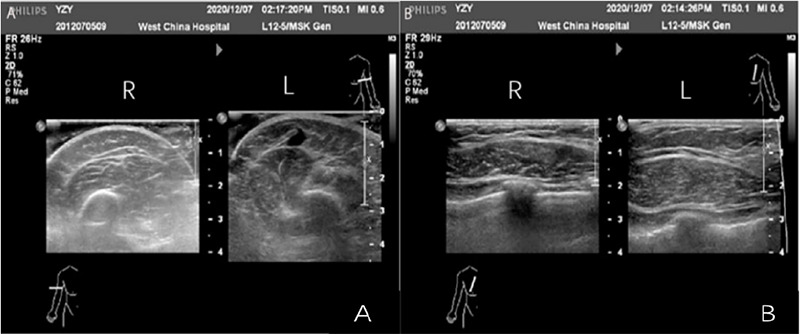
Ultrasound findings of the left sking and muscle of the left hand and chest.
